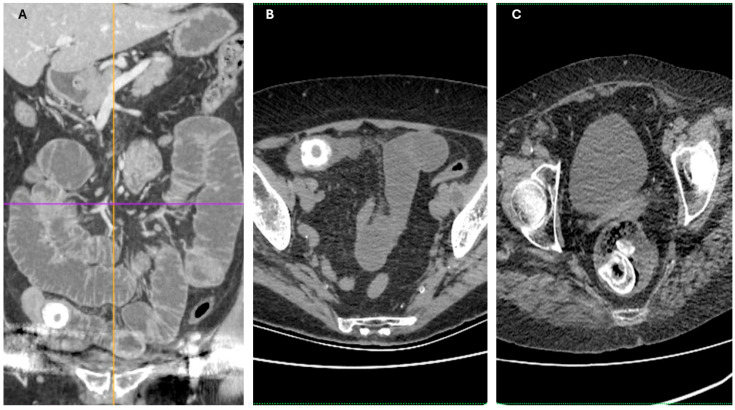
Distal migration of enteric gallstones in biliary ileus. (A,B) Coronal and axial CT images depicting a highly radiopaque enteric gallstone located in the projection of the colon-biliary ileus. (C) Axial CT section showing a radiopaque enteric gallstone in the projection of the rectal ampulla, indicating distal migration—biliary ileus. Acquisition with 5 mm slice thickness and 3 mm reconstruction. Images have been cropped and zoomed for optimal visualization while maintaining the original scale; a 10 mm scale bar is in-cluded in each panel.
